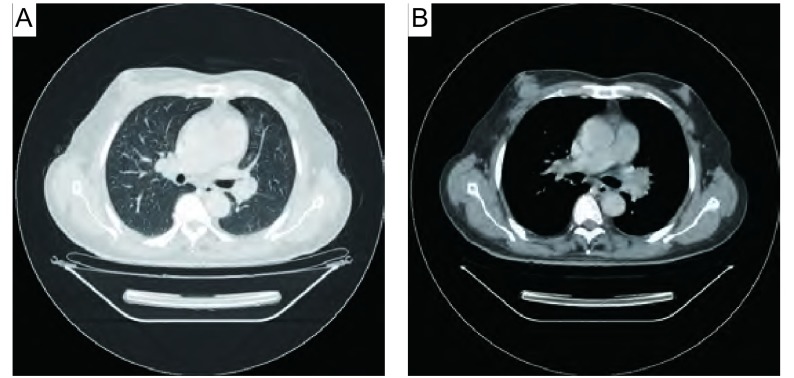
胸部CT。A：（平扫）肺窗示左肺门增大；B：（增强扫描）纵隔窗示左肺门见一软组织密度肿块，轻度强化。 Chest CT. A: (plain scan) lung window: Chest CT scan shows the left hilar increases; B: (enhanced scan) mediastinum window displays: There is a soft tissue density mass in the left hilar, and it is mild enhancement.
